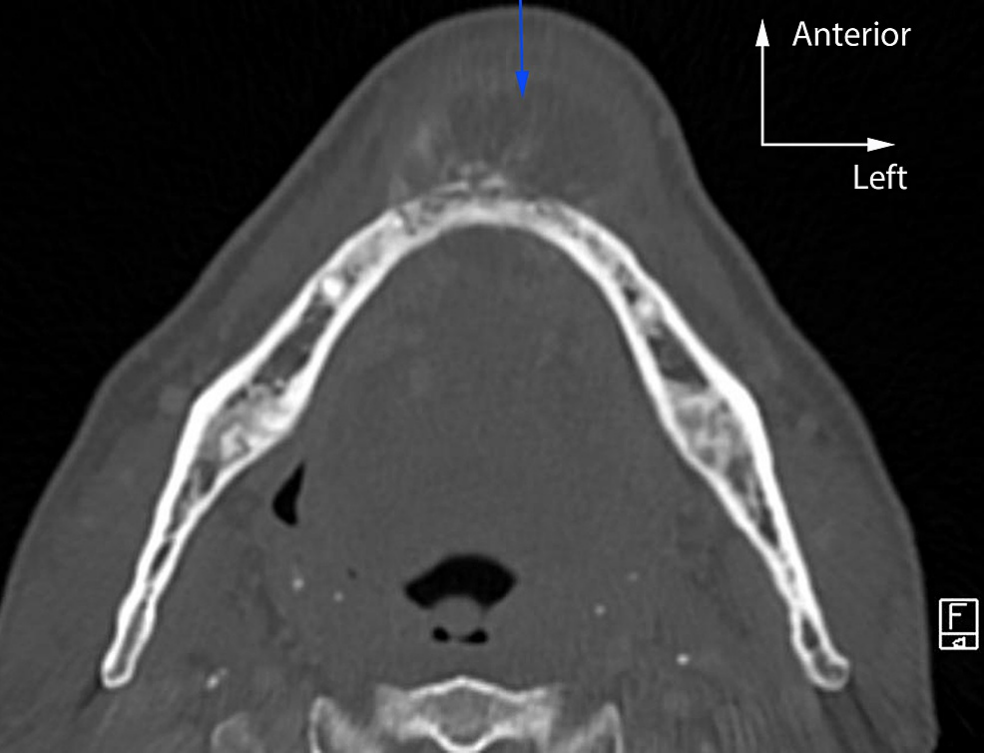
Computed tomography scan of the 38-mm-long axis lesion in the symphyseal region.
